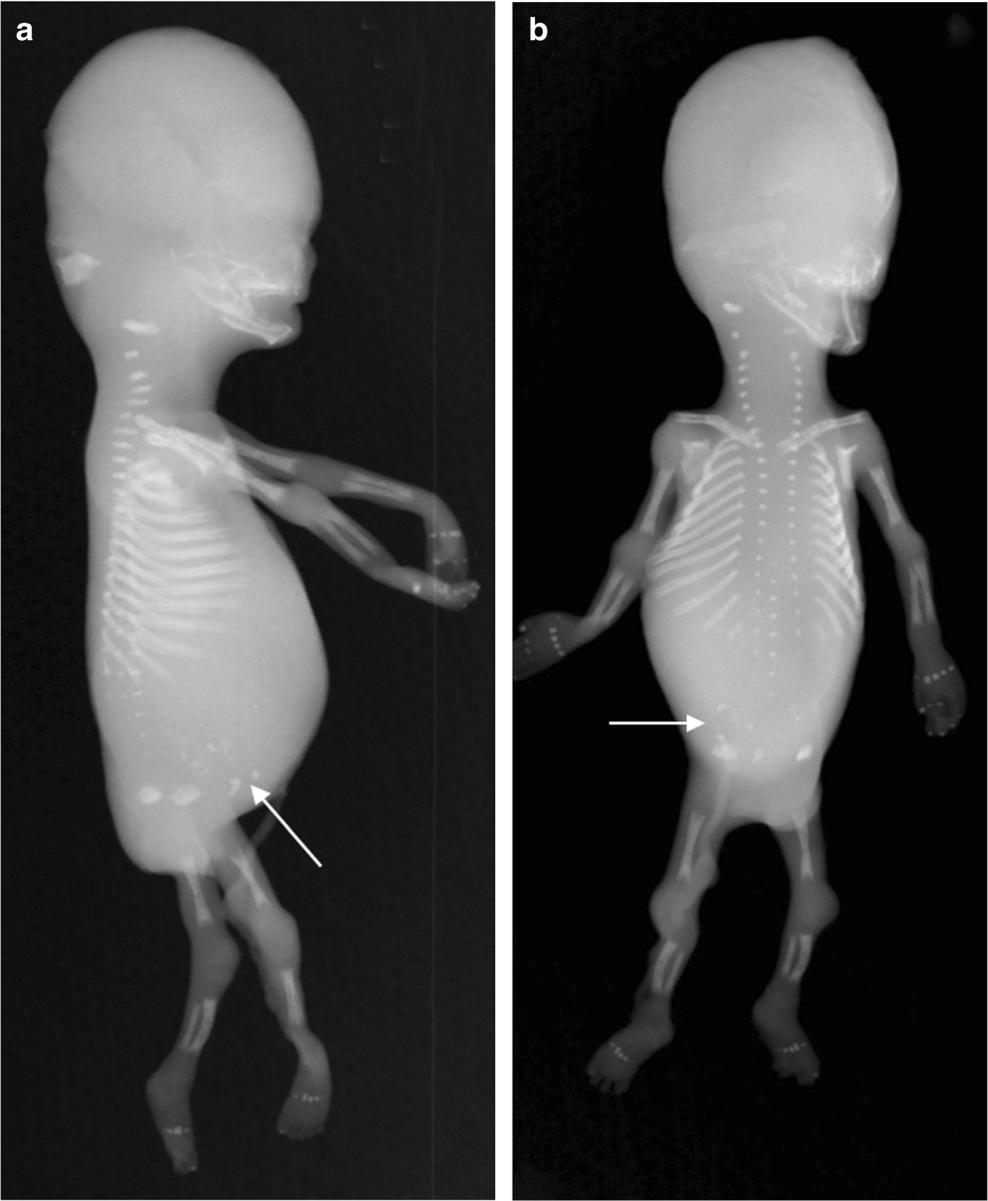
Normal ossification of the fetal skeleton of a male fetus at 11 weeks’ gestation. a,b Radiographs in lateral (a) and anteroposterior (b) projections show absent ossification of skull vault, cervical, thoracic and sacral vertebral bodies, and ischia and pubic bones. This is normal for the gestational age (the pelvic calcification [*arrows*] is probably within the bowel)
